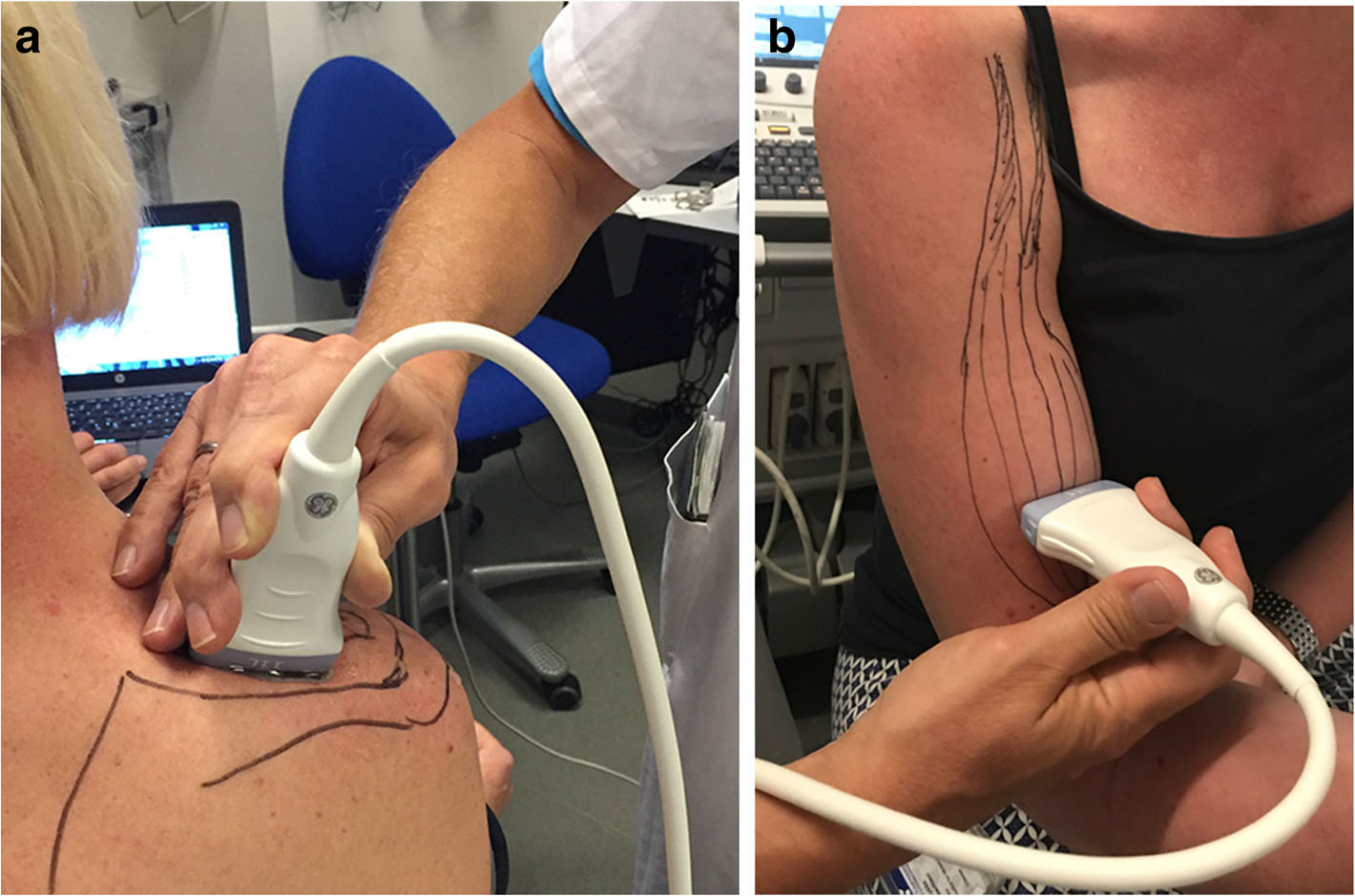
2D speckle ultrasound set-up. a, b, Transducer position to visualize the supraspinatus (a) and biceps (b) muscles
